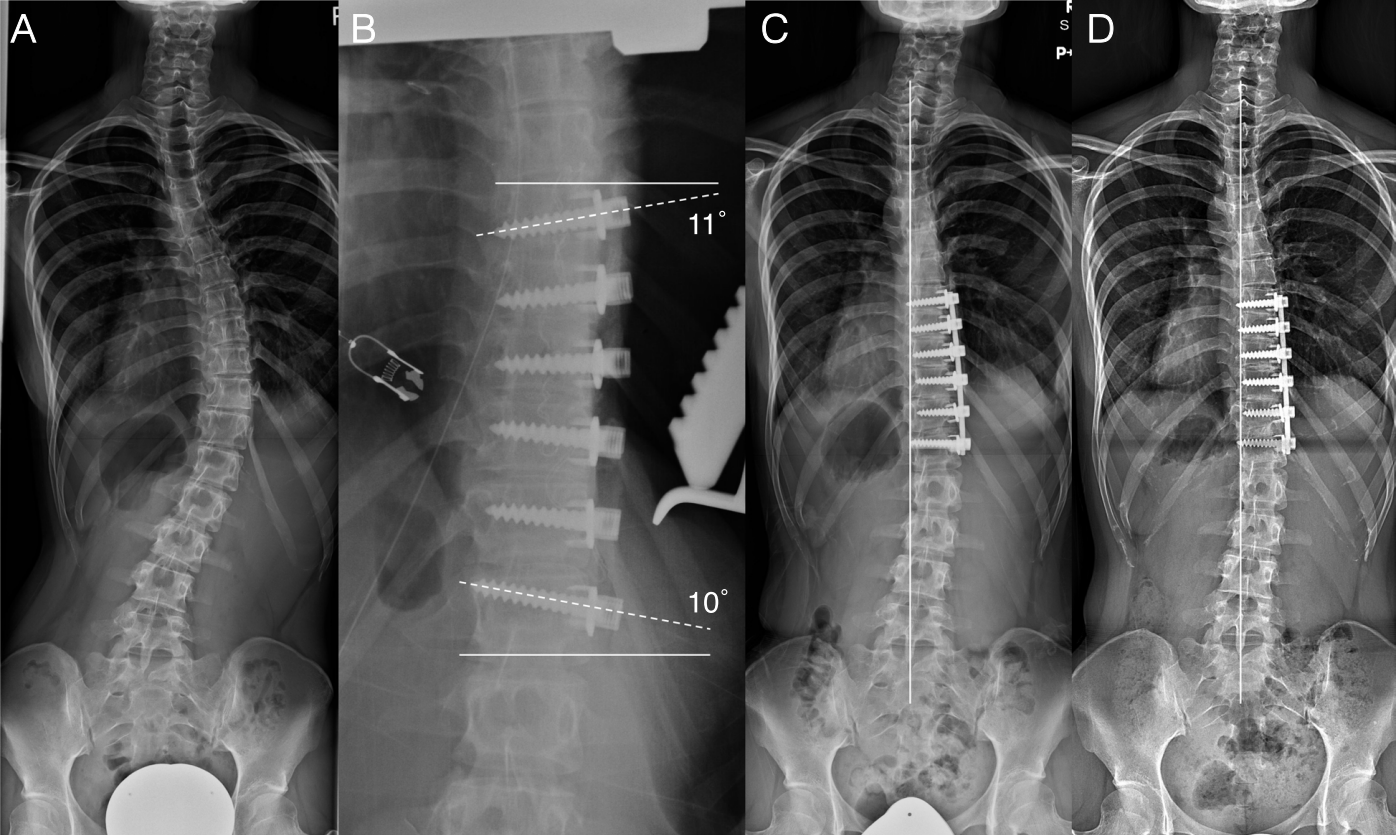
Case example of a 16-year-old female patient with Lenke 1 AR type curve. A before surgery, Cobb angle of the main thoracic curve is 47°. B intraoperative radiograph, the screw angle is 21° (i.e. angle formed by the screw axis and the upper endplate in the UIV is 11°, and the angle formed by the screw axis and the lower endplate in the LIV is 10°. The line represents the slope of endplate, and the dotted line represents the screw axis.). C at 1 month after surgery, anterior surgery has achieved overcorrection with the UIV-LIV Cobb angle of -11°, and FDUV-CSVL is 18 mm. The line is the central sacral vertical line. D at 4 years after surgery, FDUV-CSVL has gradually decreased over the postoperative period to 13 mm. The line is the central sacral vertical line
